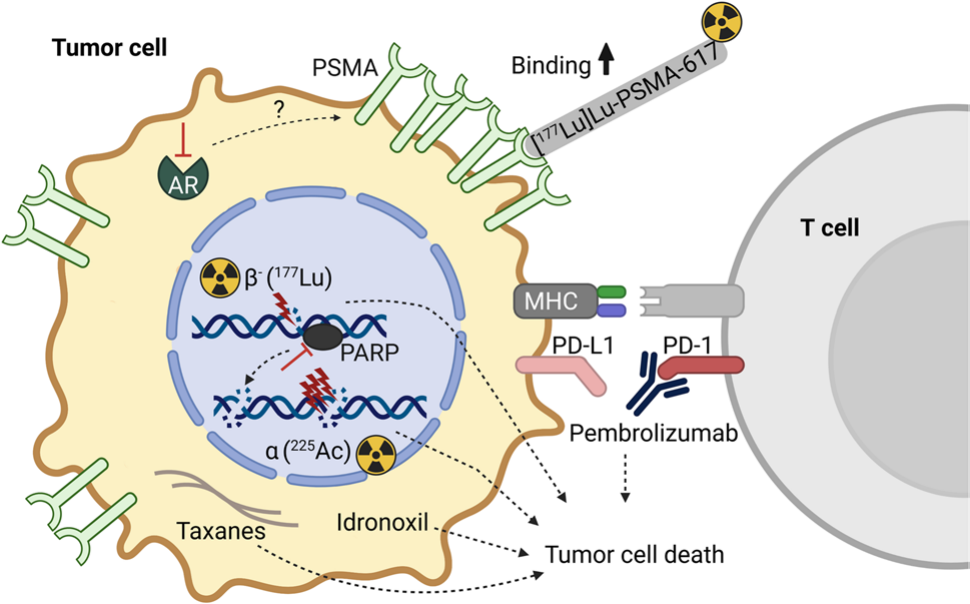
Modes of action of therapeutic agents for late-stage prostate cancer that are under evaluation for a combination therapy with [^177^Lu]Lu-PSMA-617. [^225^Ac]Ac-PSMA RLT is one of the most promising options for mCRPC patients that experienced [^177^Lu]Lu-PSMA RLT failure. Alpha particles exhibit a higher LET than *β*^−^ particles, thus, causing denser DNA damage in the form of DSBs. For [^177^Lu]Lu-PSMA RLT-naïve patients, immunotherapy with pembrolizumab emerges as a therapeutic modality with high potential in the combination with the radiopharmaceutical. Pembrolizumab binds to PD-1 receptors on T cells and abrogates the immunosuppressive interaction between immune checkpoint proteins on T cells and tumor cells. This leads to an immune attack on the tumor cells in response to recognition of neoantigens presented on major histocompatibility complexes (MHC). The genistein-derivative idronoxil facilitates cell death and led to a longer OS in combination with [^177^Lu]Lu-PSMA-617 as compared to the monotherapy with the latter. Other potentially radiosensitizing candidates are the taxane chemotherapeutic cabazitaxel and PARP inhibitor olaparib that are investigated in clinical trials. Taxanes interfere with microtubule dynamics, thereby, preventing the normal process of mitosis. PARP inhibitors suppress the sensing and repair of DNA SSBs which leads to the formation of DSBs. It remains to be investigated whether ARPI-induced PSMA upregulation, and thus increased binding capacity for [^177^Lu]Lu-PSMA-617, can translate into a higher efficacy of the radiopharmaceutical therapy. Another question is whether patients that are already receiving ARPIs can benefit from the approach. *Created with BioRender.com*
